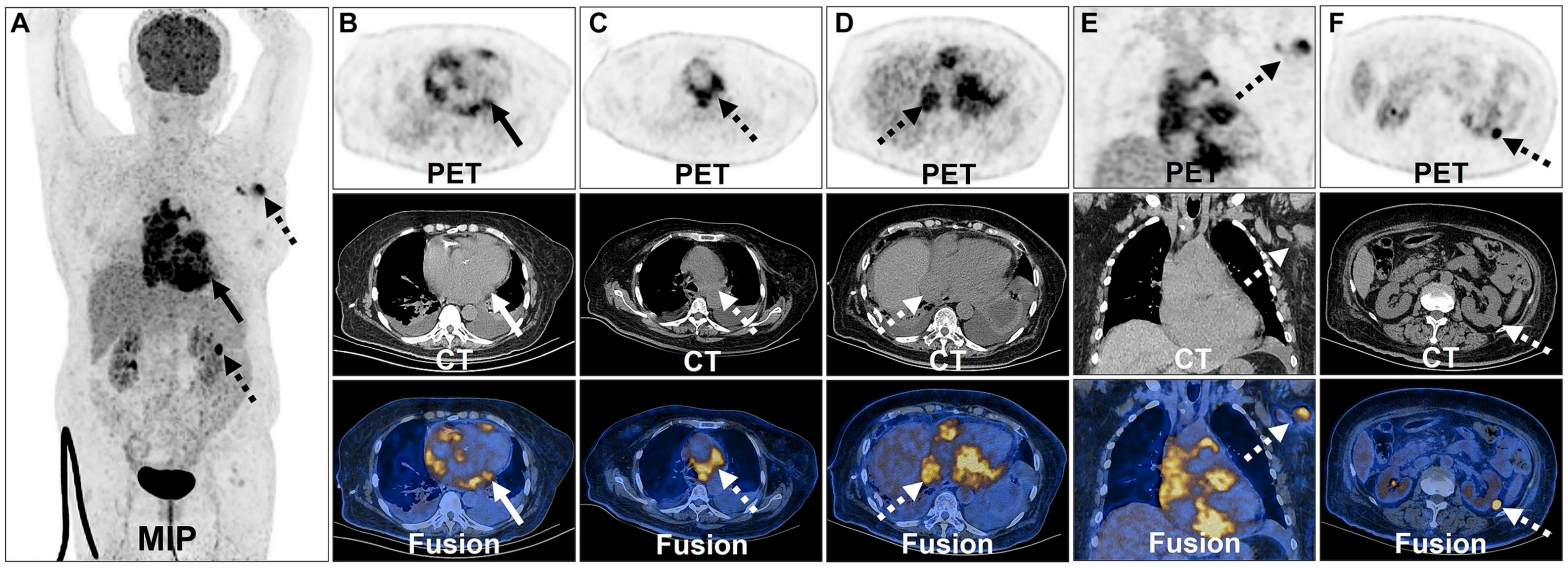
^18^F-FDG PET/CT images of PCL. The anteroposterior 3-dimensional maximum intensity projection image (MIP) demonstrated FDG-avid lesions on the heart, pericardium (long arrows) and multiple lymph nodes (dash arrows) (A). Transverse and coronal images showed multiple nodular soft tissue density lesions in the heart and pericardium exhibiting increased FDG metabolism (B). Enlarged lymph nodes were identified in the mediastinum displaying heterogeneous FDG uptake (C). The supradiaphragmatic segments of the inferior vena cava exhibited irregular morphology with localized nodular changes and increased FDG metabolism in the surrounding area (D). Enlarged lymph node was identified in the left axilla displaying heterogeneous FDG uptake (E). Enlarged lymph node was identified near the left kidney displaying heterogeneous FDG uptake (F).
